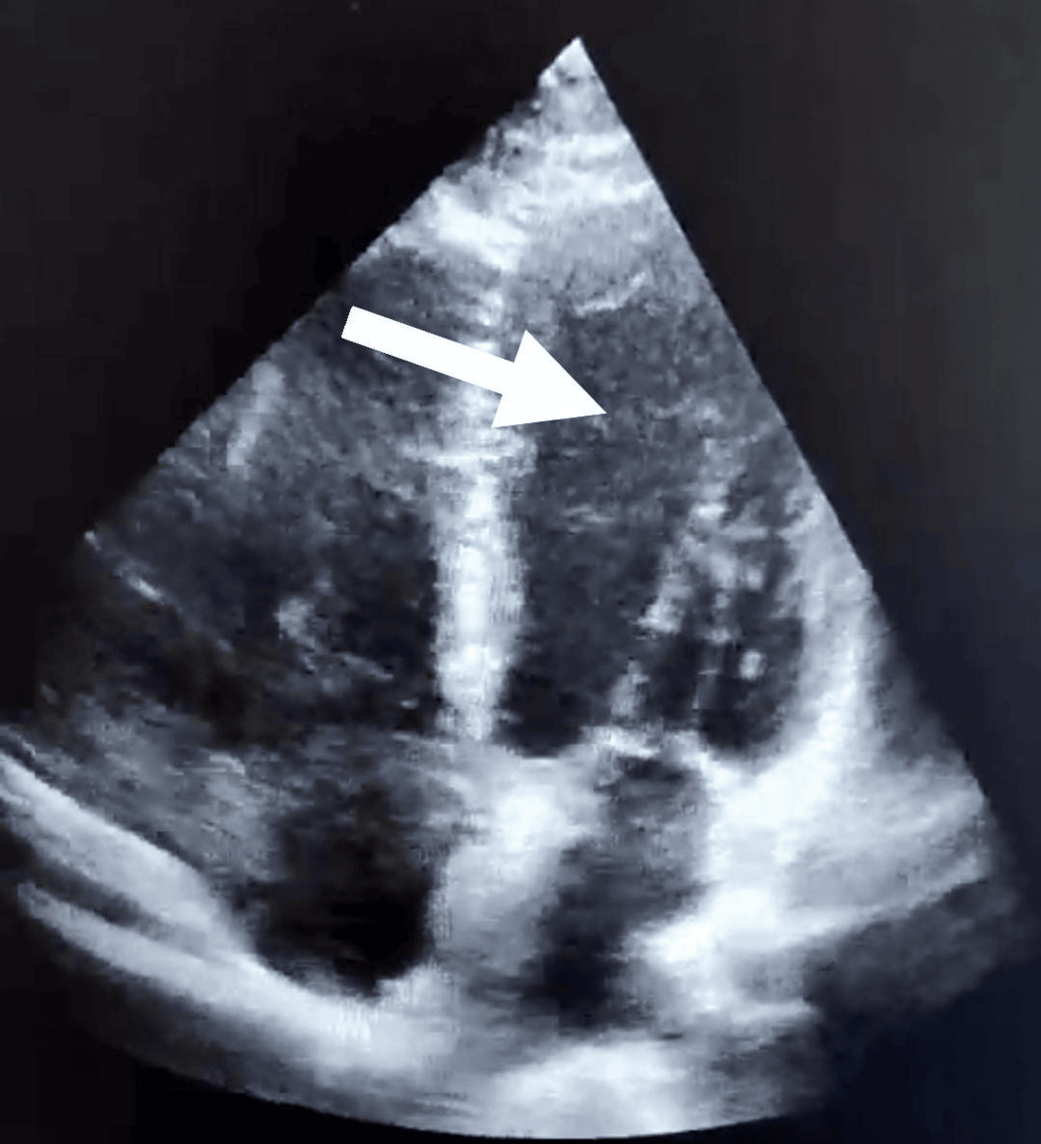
Follow-up transthoracic echocardiography showing the normalization of cardiac dimensions (left ventricular end-diastolic diameter of 52 mm) and improved systolic function (ejection fraction of 60%) with no evidence of hypokinesis.
